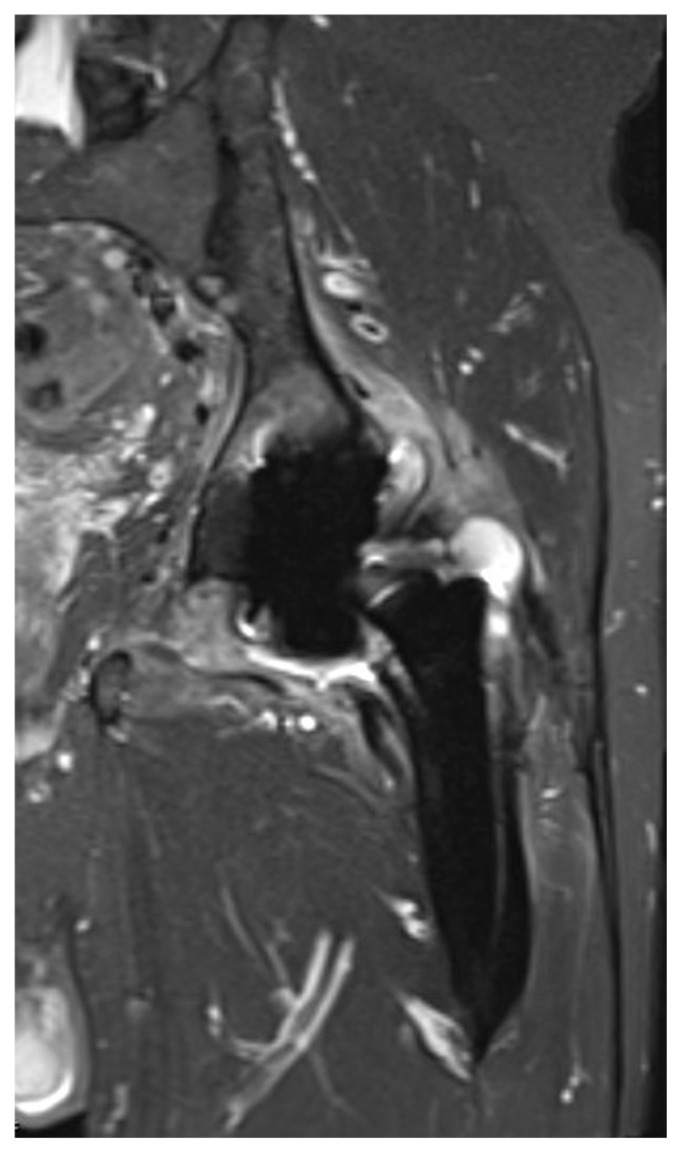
Coronal MRI of the left hip demonstrating signs suggestive of PJI, including a large, decompressing joint effusion, significant capsular enhancement, and extensive periarticular muscle edema. The presence of bone marrow edema at the metal-bone interface (Gruen zones) further suggests septic loosening of the components.
